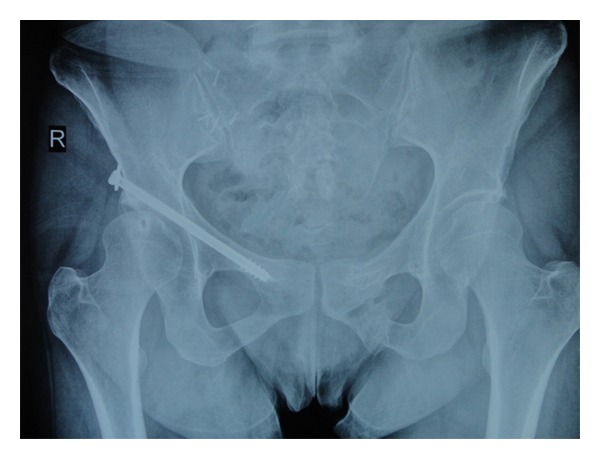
X-ray after one year of operation showing the good clinical outcome.
